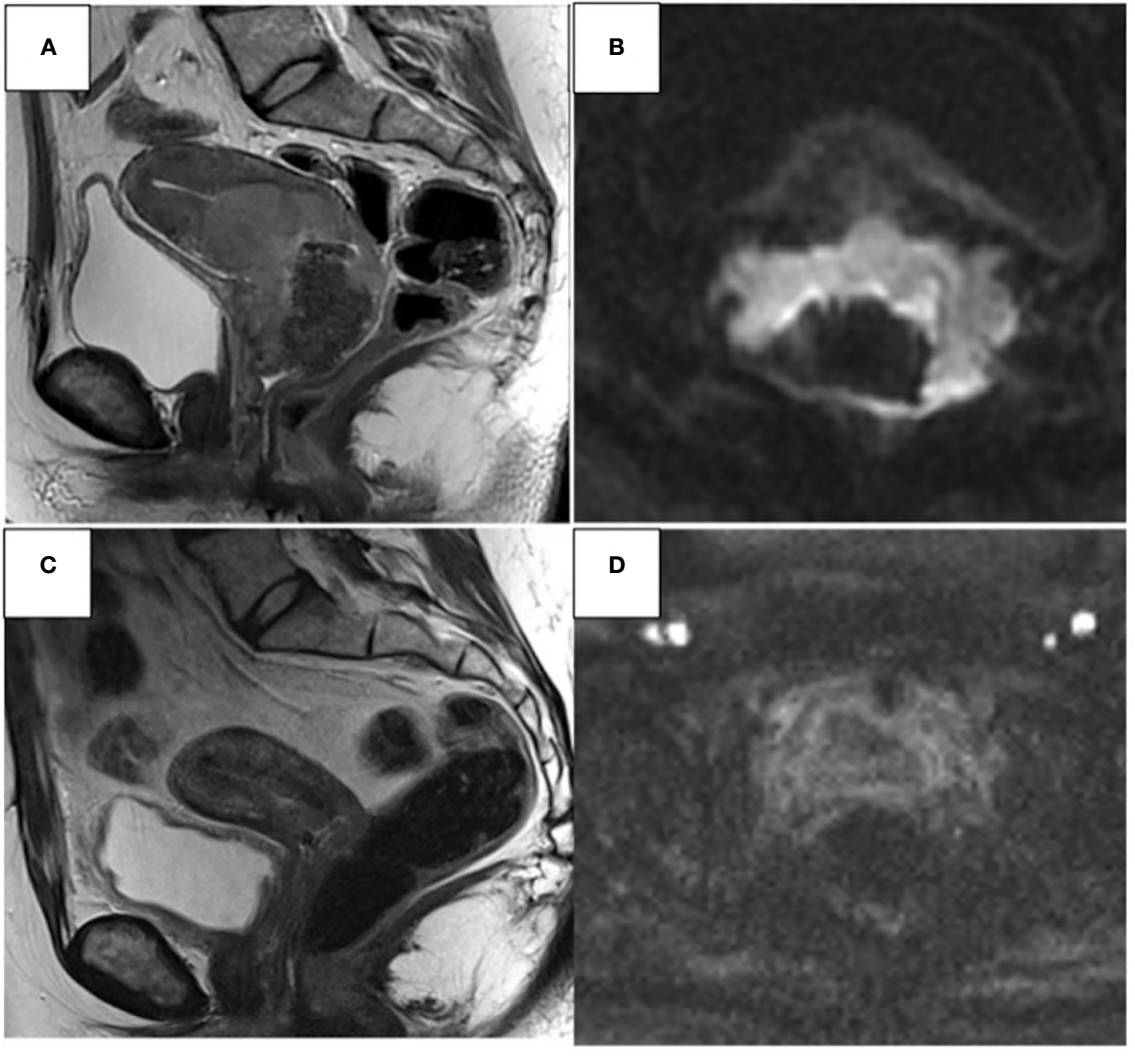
37- year-old woman. Sagittal T2W and axial DWI MR images show CC infiltrating both parameters, the upper 1/3 of the vaginal canal, the uterine body, and the left ureter (A, B). Complete response (C, D) significant post-CHT reduction of heteroplastic tissue (around 90%).
